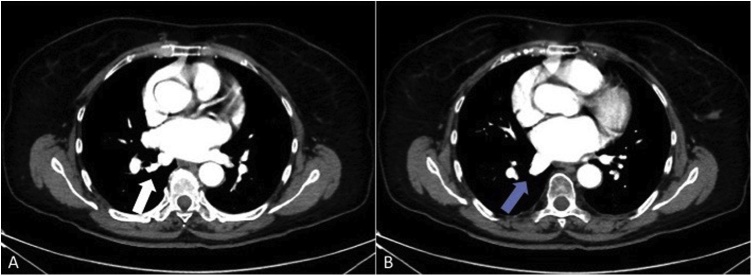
(A and B) Mediastinal window of chest CT. The radiologic images show the right inferior pulmonary vein formed by the hilar union of superior (white arrow) (A) and common basal veins (blue arrow) (B) from the lower lobe.
